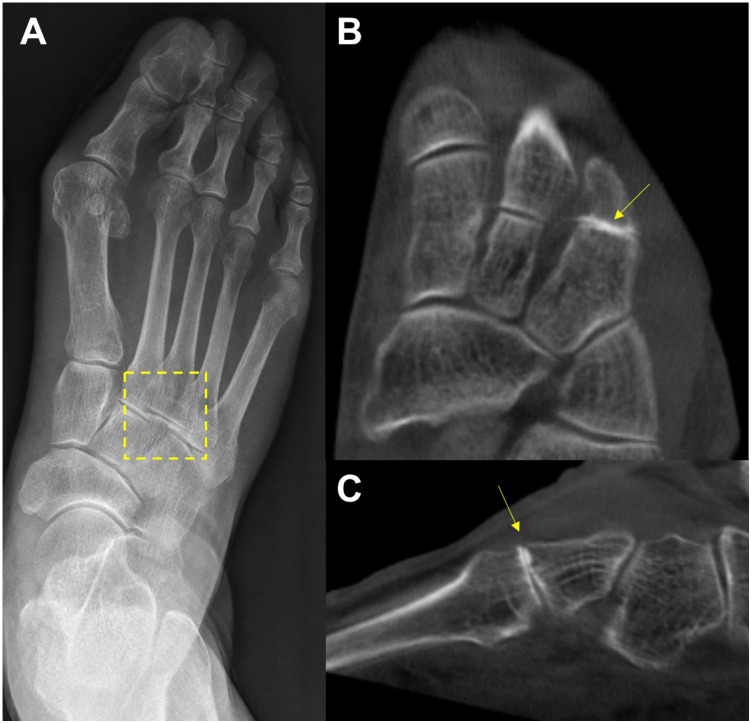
In the plain radiograph, osteoarthritis of the third tarsometatarsal (TMT) joint is not clearly visible (A, See box area); however, weight-bearing computed tomography (WBCT) reveals distinctive joint space narrowing and sclerotic changes (B,C; See arrow).
